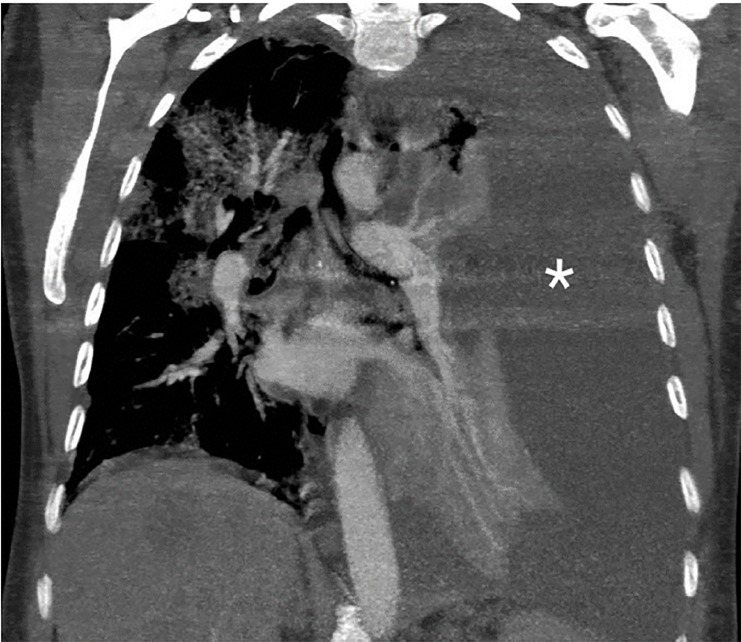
Thoracic computed tomography angiography coronal view with complete left hemithorax pleural effusion (*).
